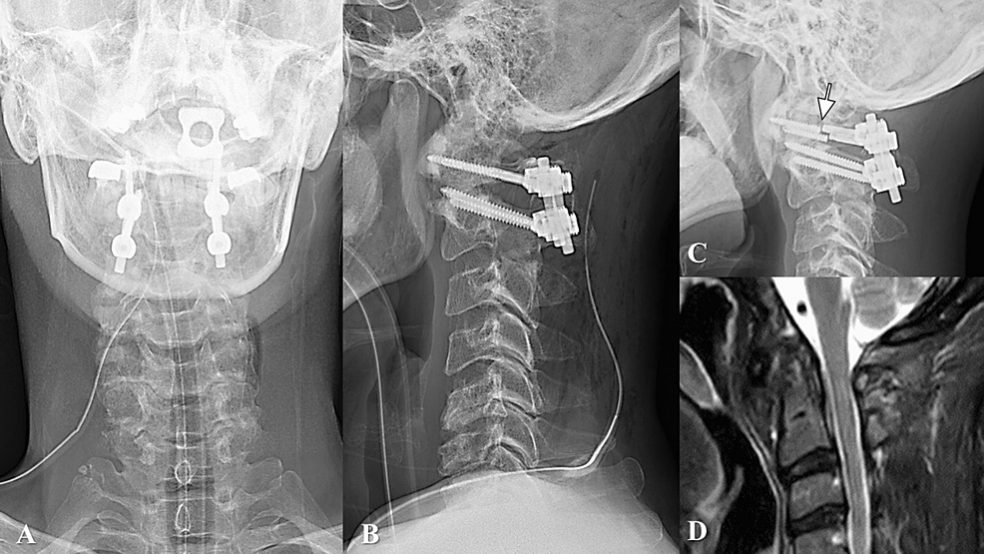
Postoperative images. A and B (plain radiography immediately after surgery). The patient underwent posterior atlantoaxial fusion surgery using C1 lateral mass screws (LMS) and C2 pedicle screws. Due to the bilateral ponticulus postics, C1 LMS were inserted inferior to the posterior arch to avoid vertebral arteries (VA) injury. All screws were inserted bicortically for stronger fixation. After instrumentation, an autologous iliac bone block was grafted between the C1 posterior arch and the C2 spinous process. C (three months post surgery). Although lateral plain radiography showed bilateral C1 LMS breakage (arrow), atlantoaxial subluxation did not recur. D (four months post surgery). Recurrent spinal cord compression was not detected in the T2-weighted MRI.
